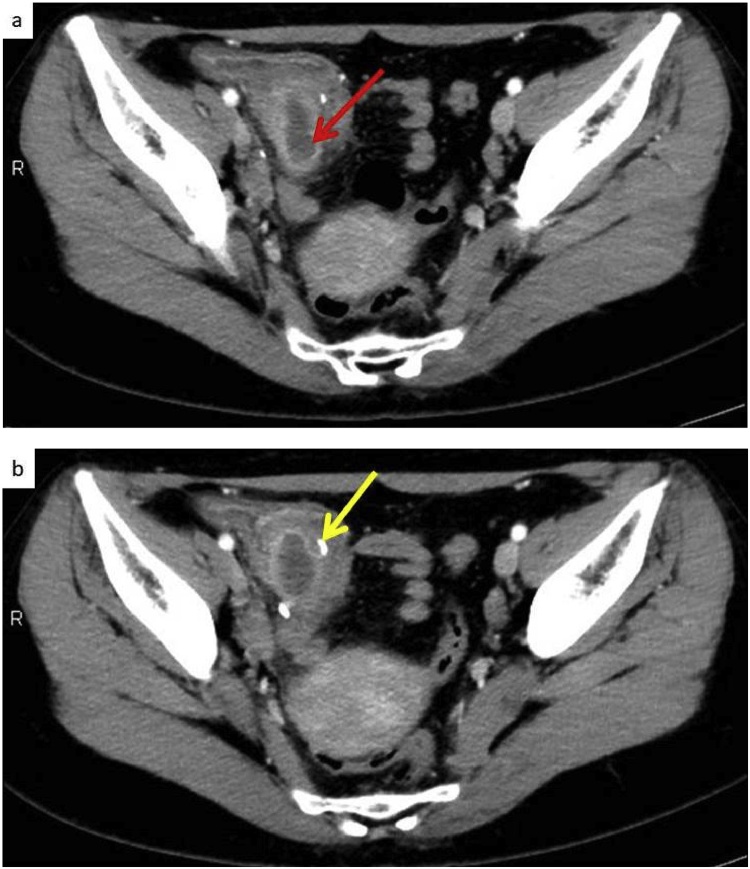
(a) CT revealed an enlarged appendix (red arrow) again. (b) The staple stump was recognized on CT (yellow arrow).
